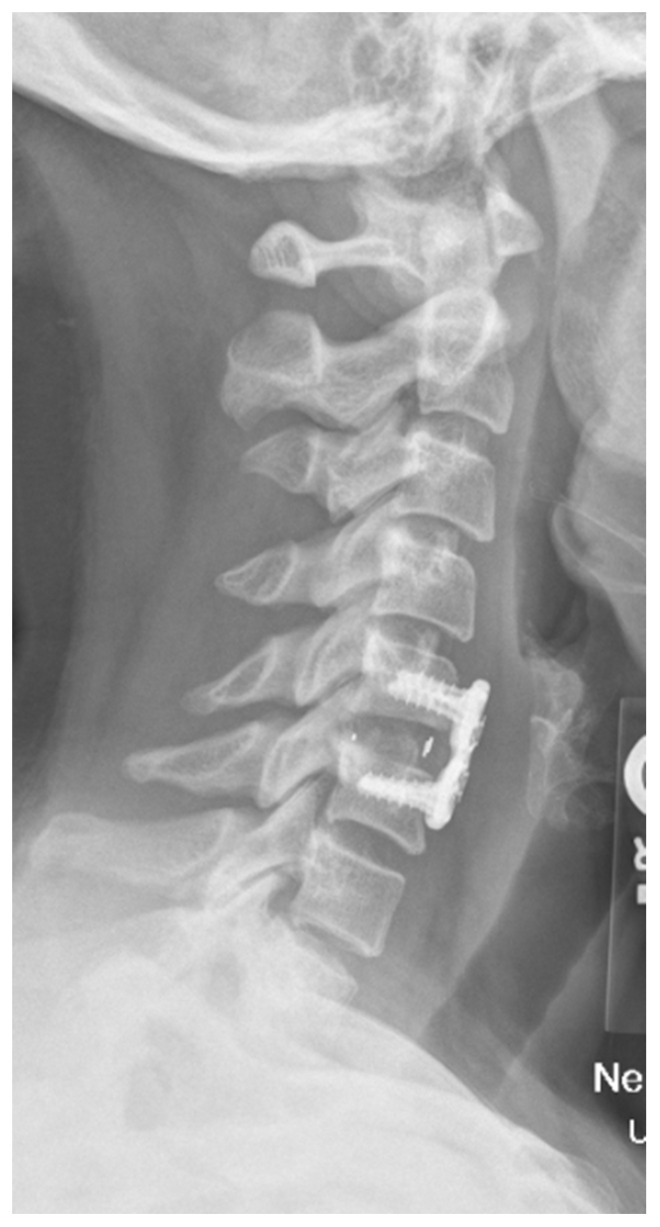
Cervical spine X-ray demonstrating polyether ether ketone (PEEK) interbody after a C5–C6 anterior cervical discectomy and fusion.
